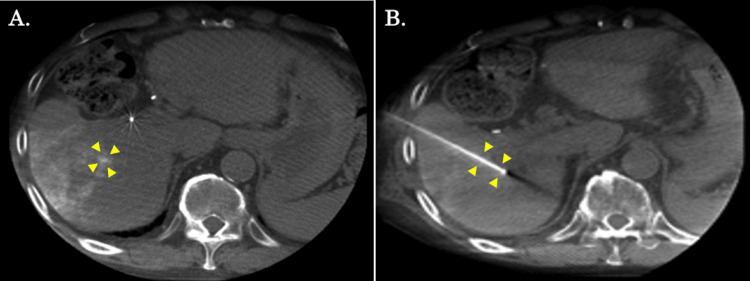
Cone beam CT findings during the radiofrequency ablation for the S8 tumor. (A) Cone beam CT shows lipiodol accumulation in the target lesion of liver S8 (yellow arrows). (B) Ablation is performed after the lesion has been pierced with a needle (yellow arrows).
